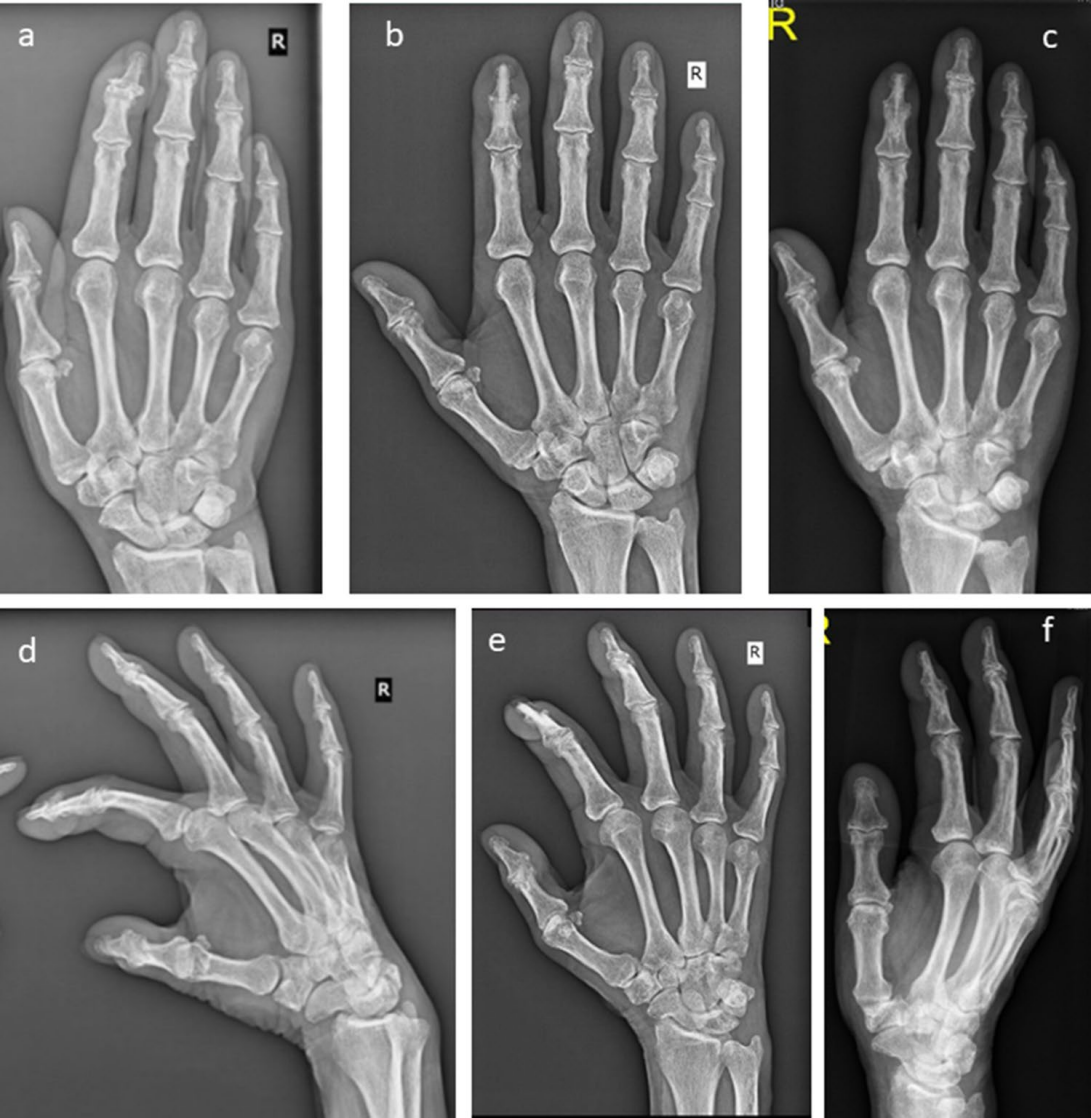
X-ray of DIP arthrodesis: a, d: pre-surgery; b, e: 6 weeks post-surgery; c, f: 1 year post-surgery, a, b, c: ap view, d, e, f: lateral view
